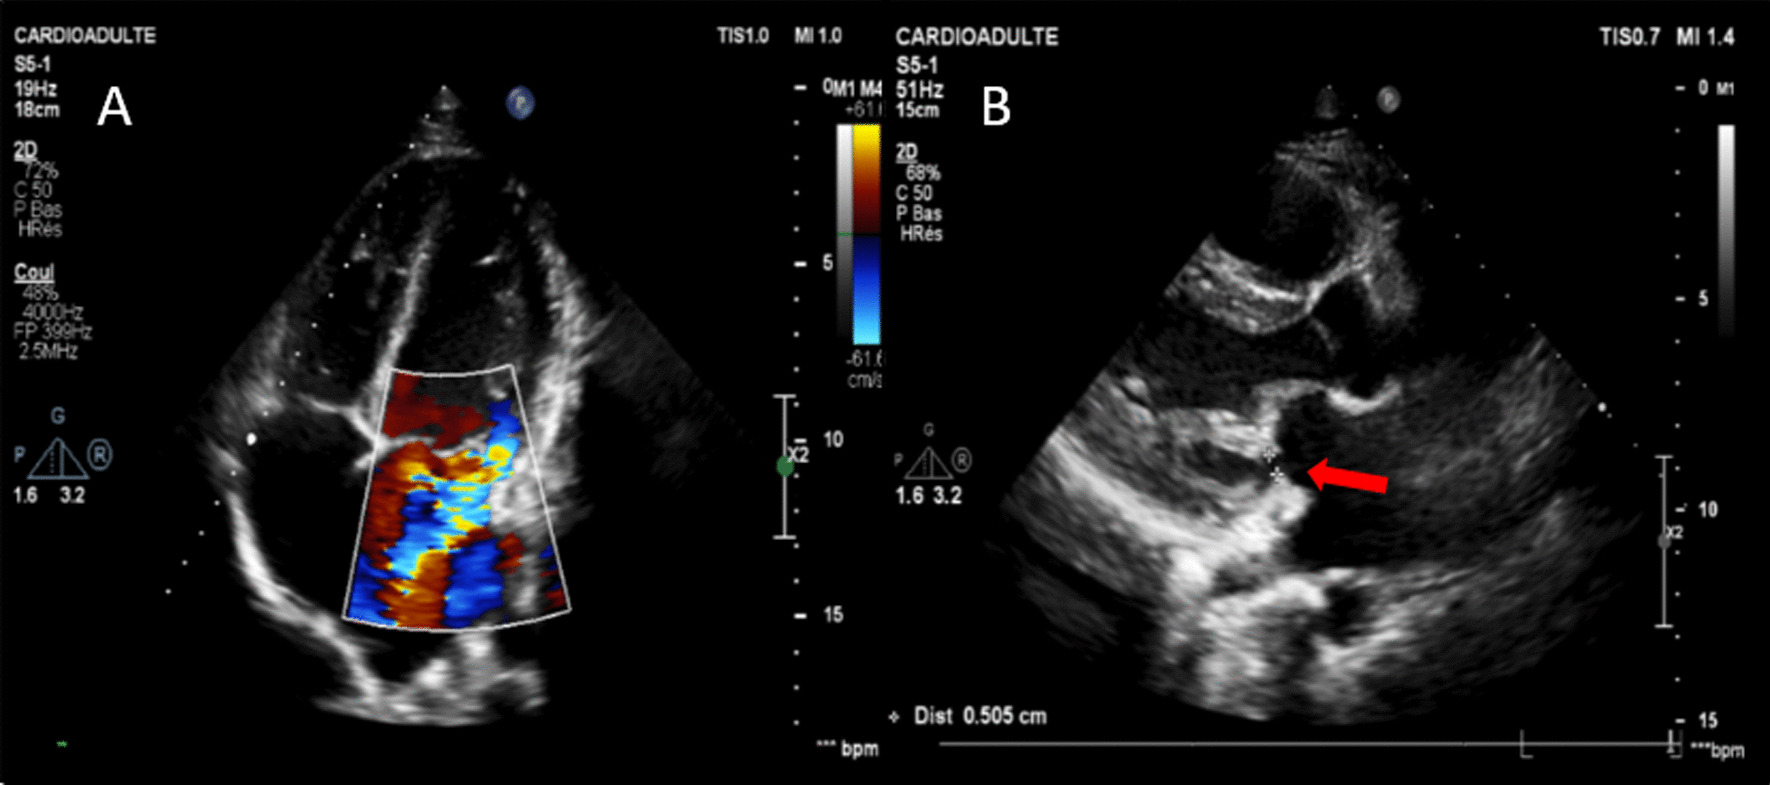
Transthoracic echocardiogram demonstrating mitral valve regurgitation; a four cavities view showing the eccentric jet of mitral regurgitation; b parasternal long-axis view showing the perforation of mitral posterior leaflet
